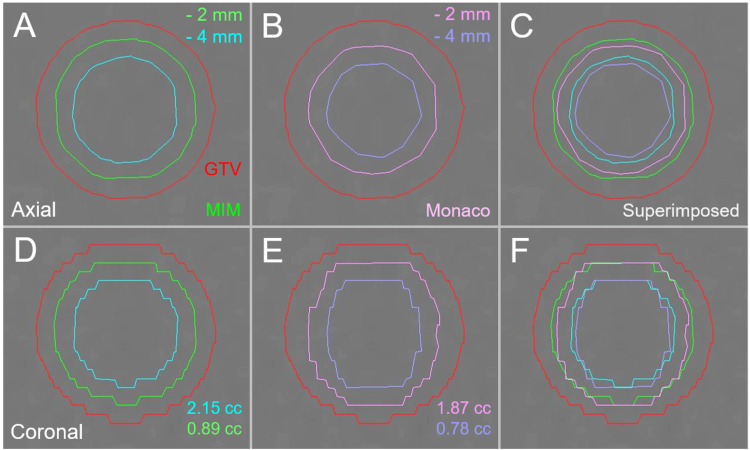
The differences in functions to reduce volume evenly between two planning systems in a 20-mm sphere model. The images show the target contours superimposed onto non-contrast-enhanced (CT) images (A-F); axial images (A-C); coronal images (D-F); by MIM Maestro (A and D); by Monaco (B and E); and the superimposed images (C and F). The differences in the volumes are equally reduced by 2 mm and 4 mm from the identical spherical model with a diameter of 20 mm. CT: Computed tomography; GTV: Gross tumor volume; GTV - X mm: Gross tumor volume evenly reduced by X mm; MIM: MIM Maestro
